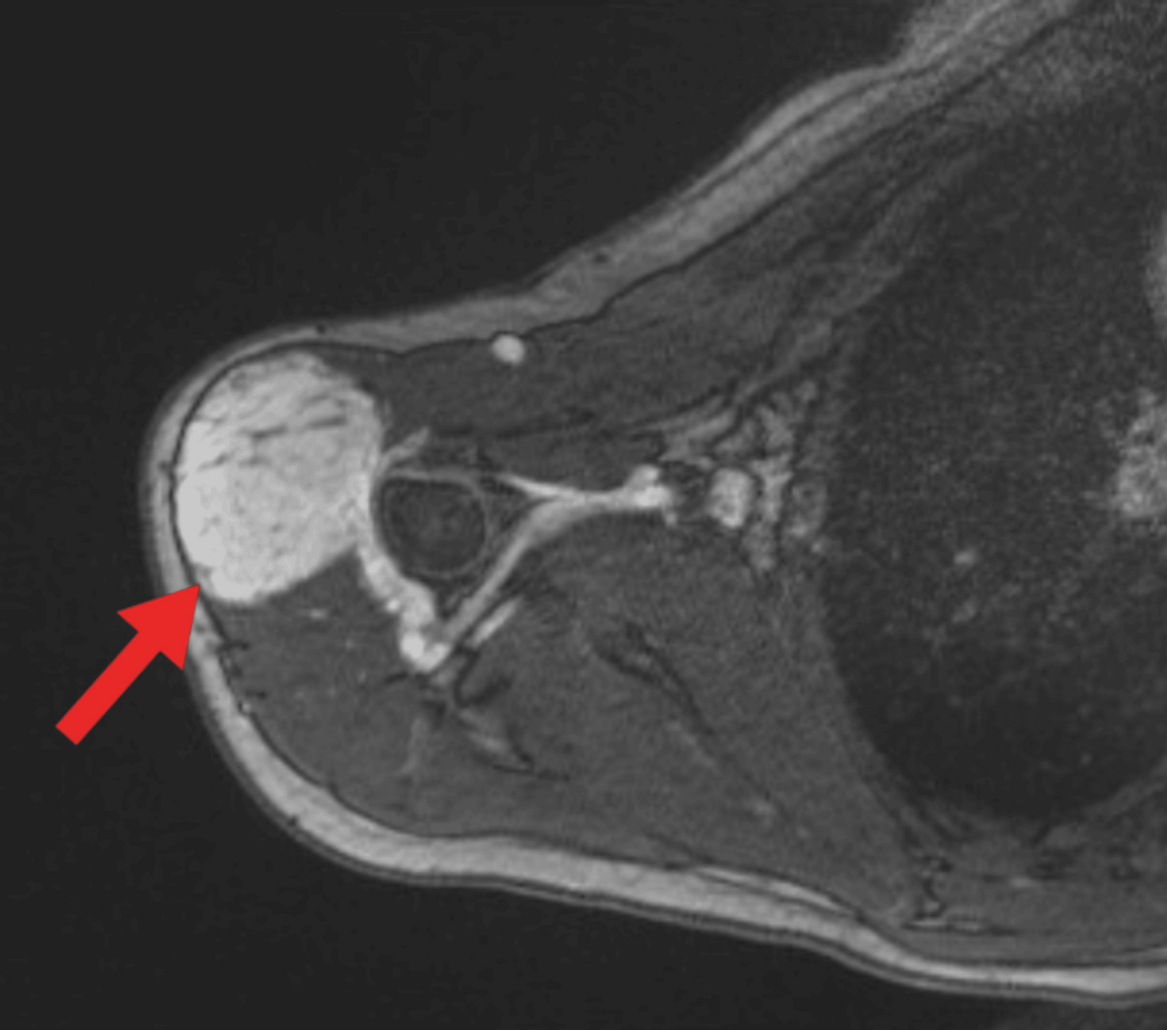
Contrast-enhanced MRI of the right humerus The red arrow points to a large fusiform area with mixed signals occupying the entire right deltoid muscle, with regions of high signal indicating fatty deposition. The lesion measured 8.5 x 4.1 x 4.7 cm, with no extension into surrounding tissues.
